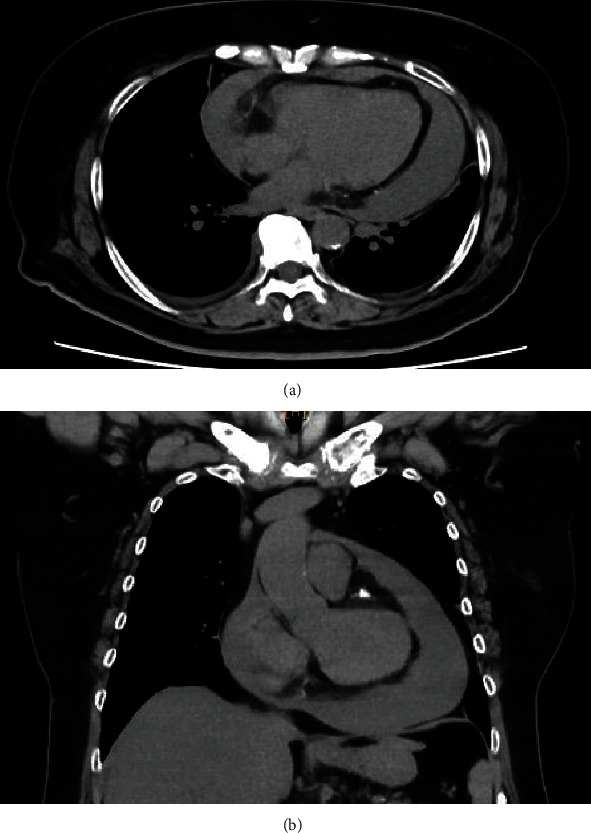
The findings of pericardial effusion before drainage and steroid therapy in computed tomography (CT) (a, b).
